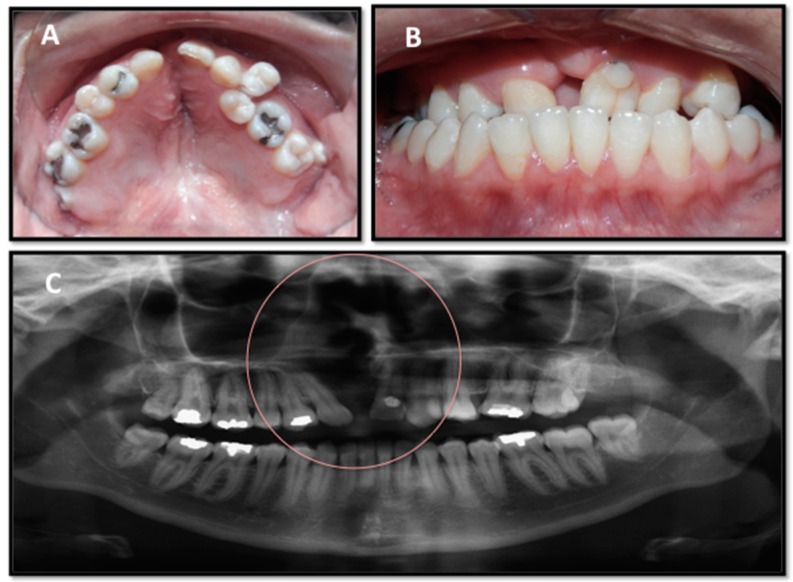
Image of a patient with unilateral cleft palate showing the different tissues involved (bone, dental organs, respiratory system and soft tissue) that need to be attended during the treatment and some malformation around the orofacial area responsible for causing respiratory and speech/language problems. Deformation of the arch and dental crowding (A), crossbite dental malposition (B), and the deviated nasal septum (C) as revealed by panoramic radiographs showing the maxillary defect (circle) (unpublished data).
